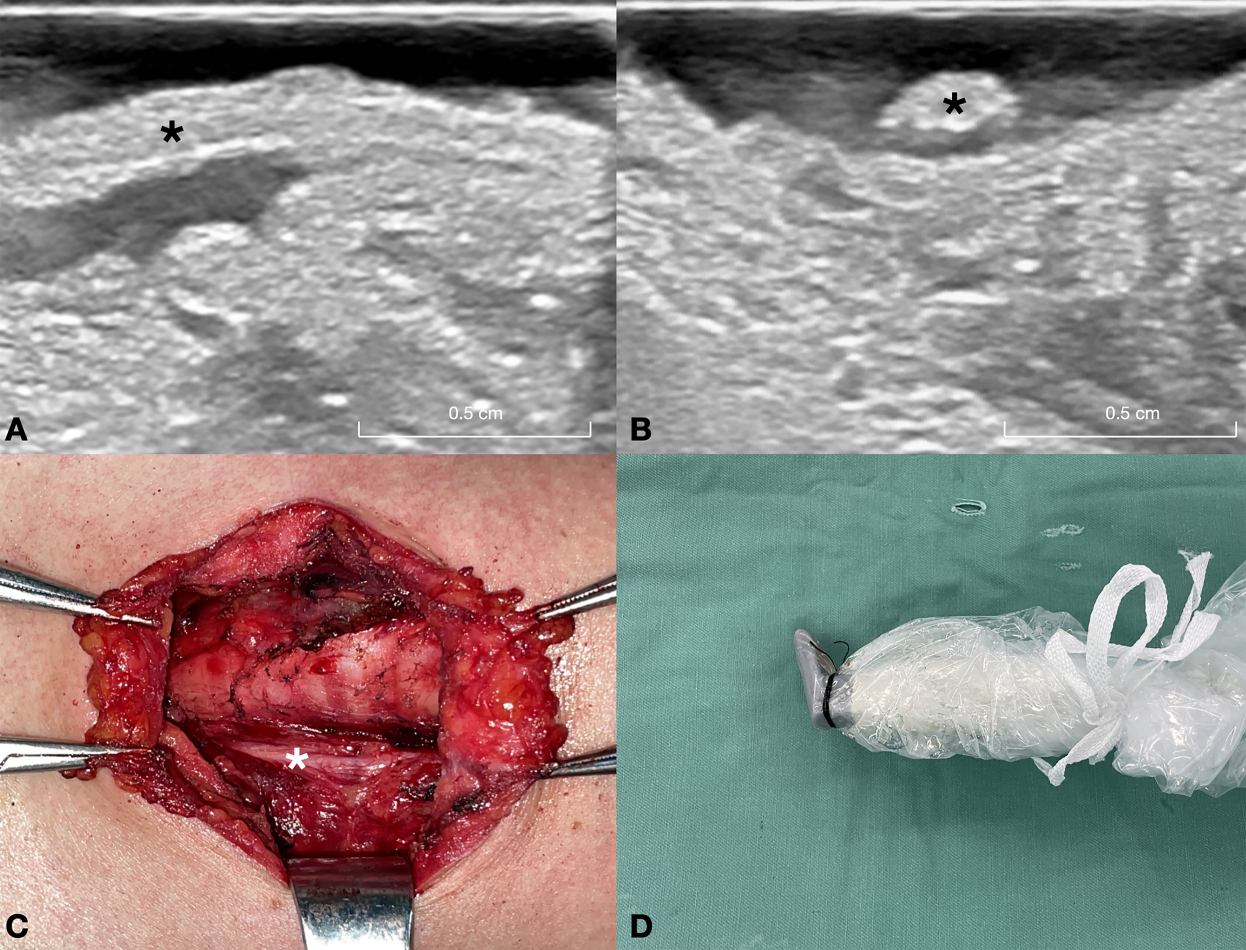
This figure depicts a 32-year-old female patient with a nodular goiter in the right lobe of the thyroid gland. (A) Long-axis ultrasound section of the normal RLN (asterisk). (B) Short-axis ultrasound section of the normal RLN (asterisk). (C) Intraoperative exposure of the normal RLN (asterisk) following thyroid gland excision. (D) Intraoperative ultrasound probe image of the normal RLN. A warm solution of physiological saline is administered into the surgical field, and the ultrasound probe is positioned above the RLN for imaging. RLN, recurrent laryngeal nerve.
